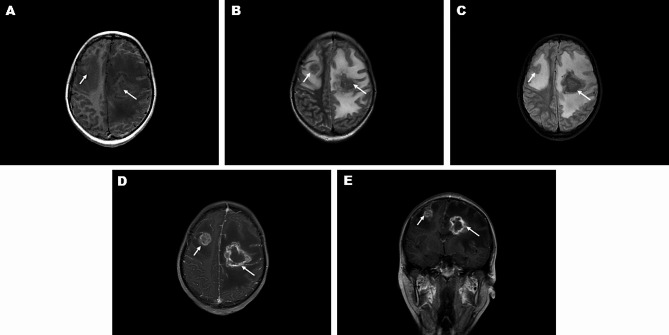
MRI Imaging Features of CAE Lesions (The patient was a 13-year-old girl with two lesions, the lesions indicated by white arrows): (A) Slightly hyperintense signals in the lesion on cross-sectional T1WI; (B) Non-uniform hypointense signals in the lesion on cross-sectional T2WI; (C) Non-uniform hypointense signals in the lesion on cross-sectional T2W-FLAIR; (D-E) Honeycomb or cauliflower-like enhancement observed at the lesion edges on contrast-enhanced MRI images
